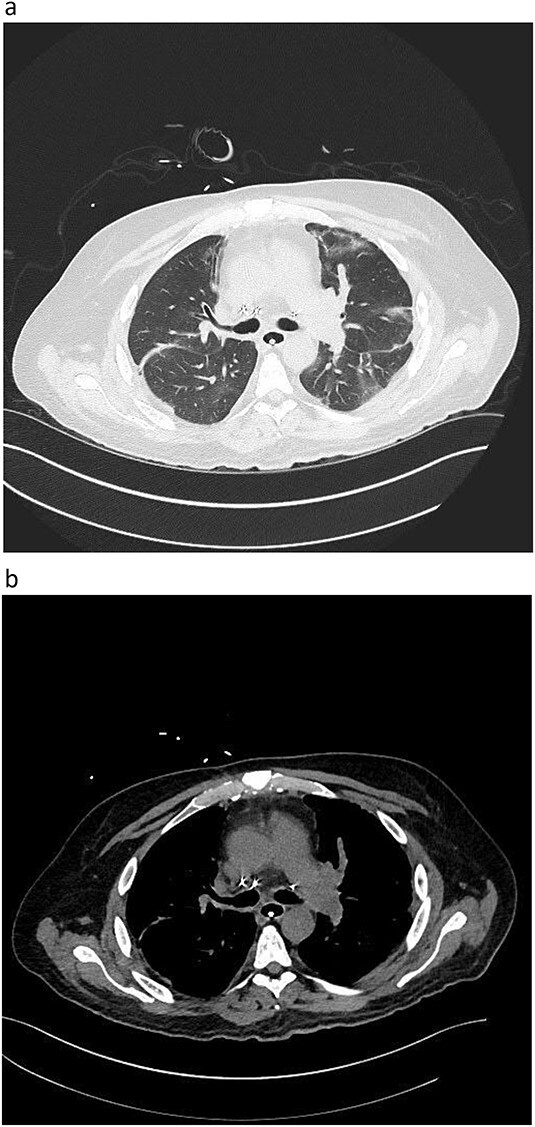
Postoperative graft CT scan: recipients had bilateral lung reduction due to over size.
